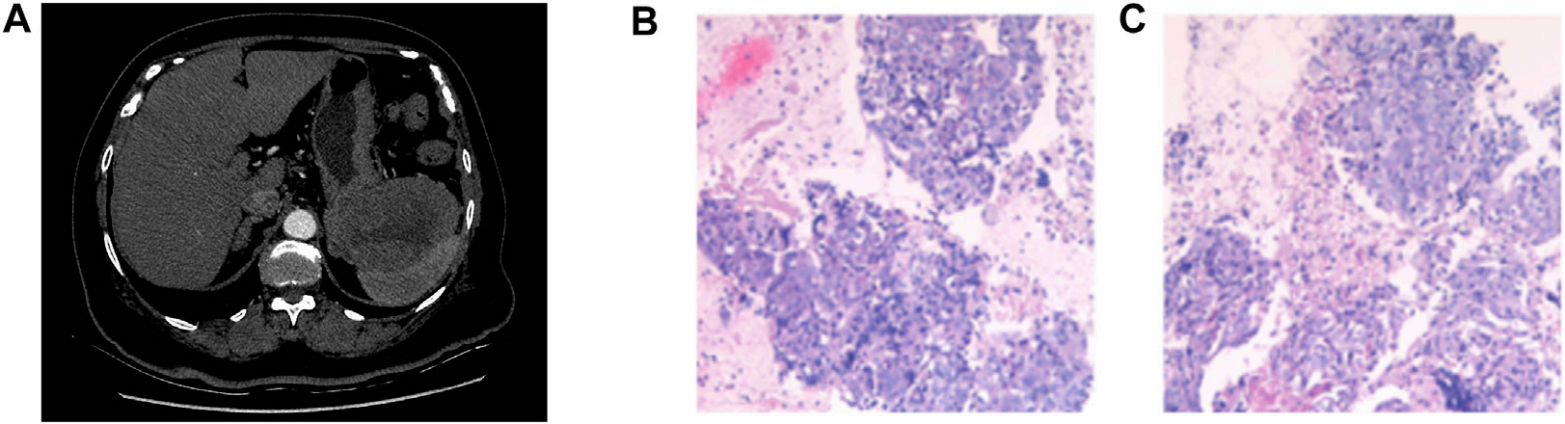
CT scan on 31 May 2019 revealed multiple masses in the gastrosplenic recess, the inferior capsule border of the spleen, and the right peritoneum (A). Pathology results revealed poorly differentiated metastatic adenocarcinoma (B, C).
